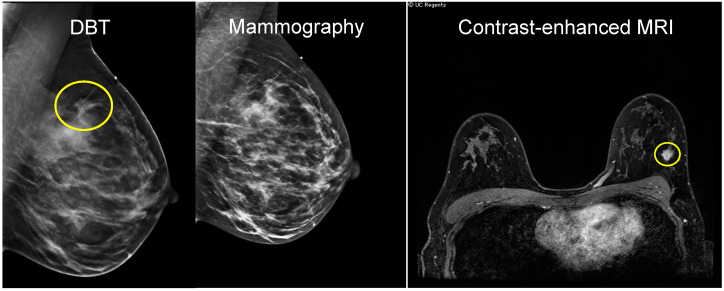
An invasive ductal carcinoma (IDC) presenting as palpable lump, best seen on tomosynthesis slices of DBT (left), with asymmetry and architectural distortion. The heterogeneously dense breast tissue obscures the finding on the 2D mammographic image (center). Ultrasound (not shown) demonstrated a hypoechoic lesion and was redemonstrated by contrast-enhanced MRI with an irregular mass measured at 1.2 × 1.1 × 1.4 cm (right).
